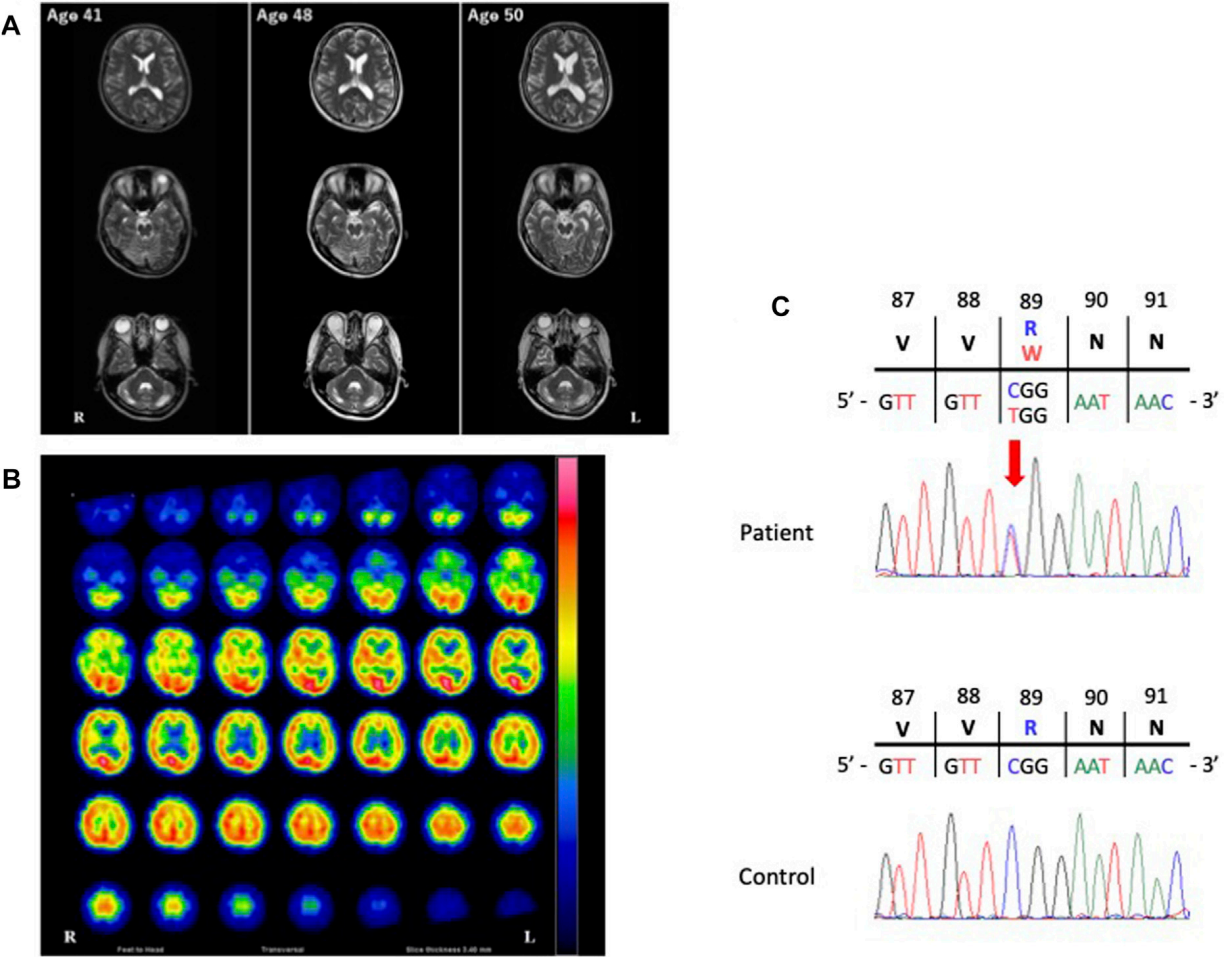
Images of the present patient and the nucleotide variant. (A), The course of T2-weighted brain magnetic resonance imaging (MRI). Longitudinal brain MRI showed progressive brain atrophy with left temporal dominance and non-progressive cerebellar atrophy. (B), Brain N-isopropyl-p-[123I] iodoamphetamine single photon emission computed tomography (^123^I-IMP SPECT). The patient’s^123^I-IMP SPECT image acquired at the age of 50 years showed hypoperfusion in the bilateral temporal lobes with left dominance and the bilateral cerebellar hemispheres. (C) Electropherogram of the region of the variant of the valosin-containing protein gene (NM_007126.5: exon 9: c.265C>T [Arg89Trp]) in our patient and an unaffected control. The location of the variant is indicated by a red arrow.
